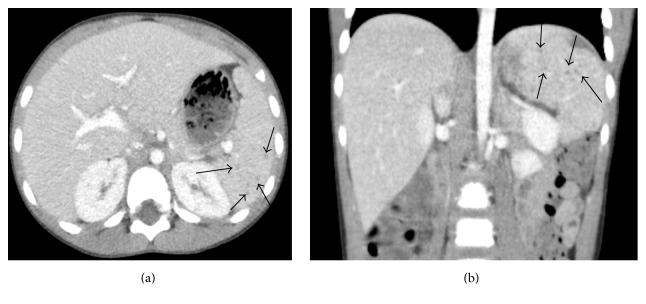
Axial and coronal contrast-enhanced CT images through the upper abdomen demonstrate an enlarged liver. The spleen demonstrates multiple small hypoattenuating lesions (arrows).
